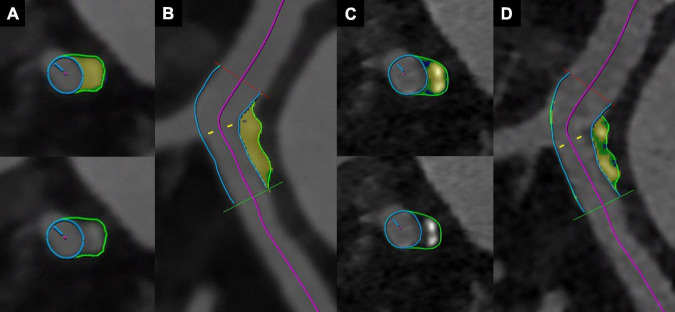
Coronary CT angiography with photon-counting detector CT of an 88-year-old male patient. Images show a mixed plaque reconstructed with a slice thickness of 0.6 mm and the Bv40 kernel (A,B), and with a slice thickness of 0.2 mm and the Bv64 kernel (C,D). Note the improved visualization of non-calcified components (blue color corresponds to lipid rich components, green color to fibrotic components, and yellow color to calcified components) at a slice thickness of 0.2 mm with the Bv64 kernel (C,D).
